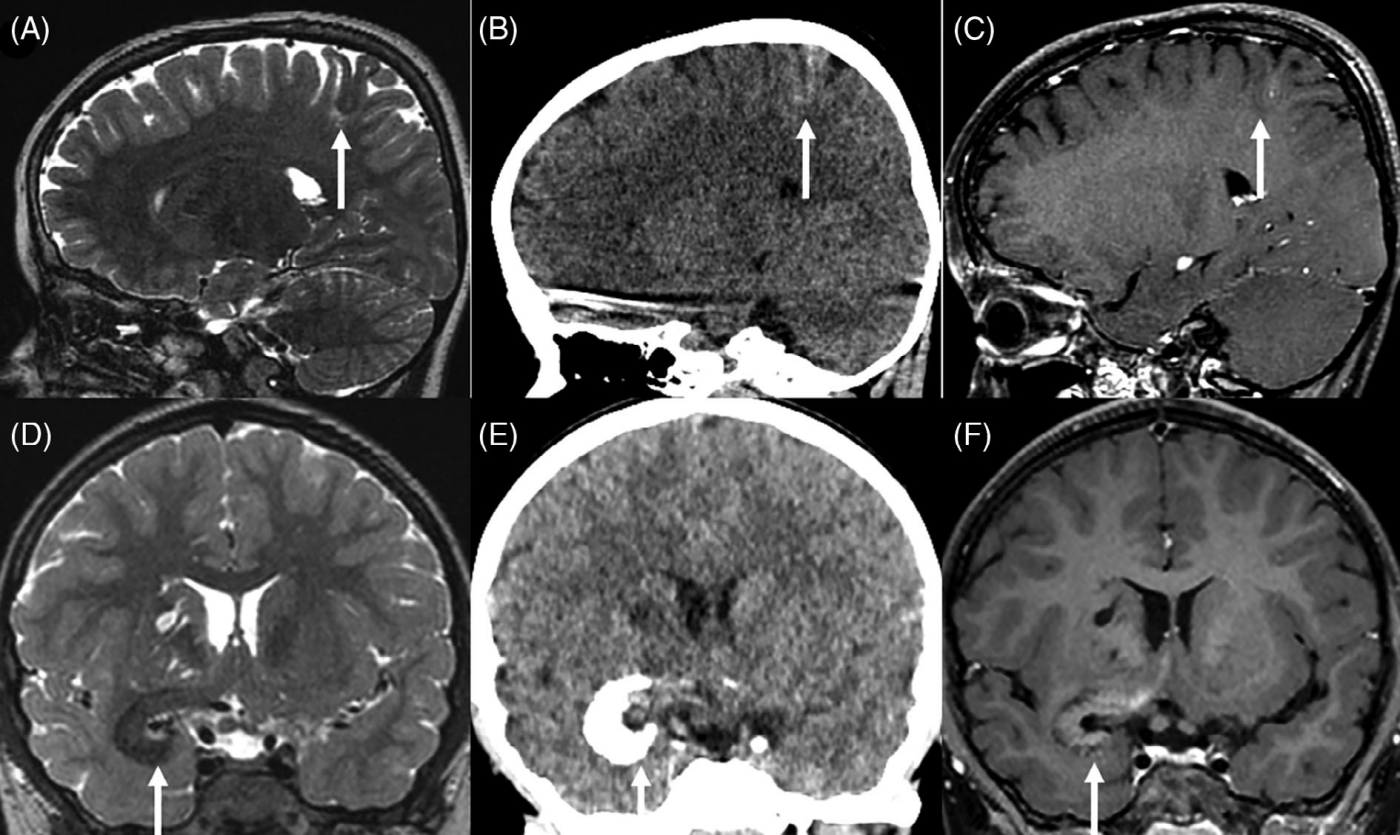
Neuroradiological features of meningioangiomatoses. Magnetic resonance imaging of patient #4, showing a cortical T2‐weighted low intensity signal with a subcortical high intensity signal (A), high density on computed tomography with microcalcification (B) and very slight contrast enhancement (C). Magnetic resonance imaging of patient #1, depicts a thick cortex with T2‐weighted low intensity signal and enlarged Virchow‐Robin spaces in the basal ganglia (D), coarse calcification on computed tomography (E) and contrast enhancement (F).
